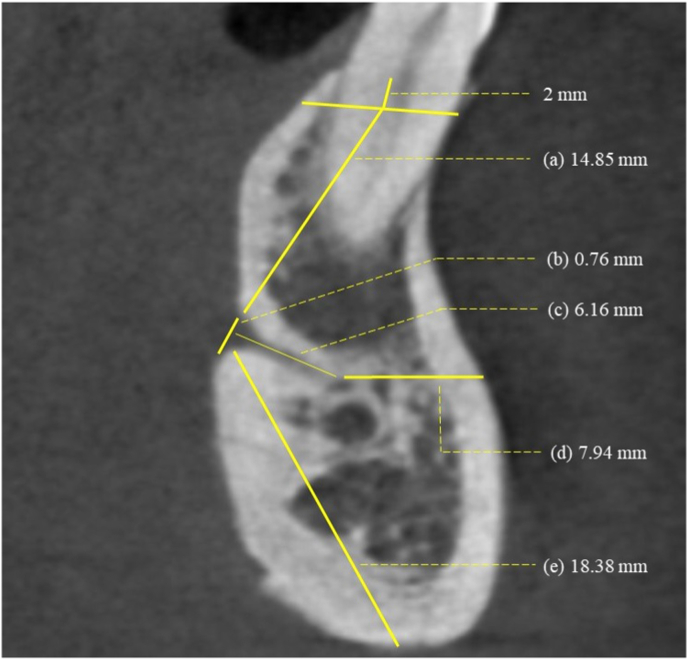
Measurements in the coronal section of the cone beam computed tomography (CBCT) scan. a. Vertical distance from 2 mm apical to the alveolar crest to the LF (Hcre). b. Diameter of the LF. c. Length of the lingual canal (LLC). d. Horizontal distance from the anterior-most point of the lingual canal to the labial cortical plate (LC-CP). e. Vertical distance from the inferior border of the mandible to the LF (Hinf).
